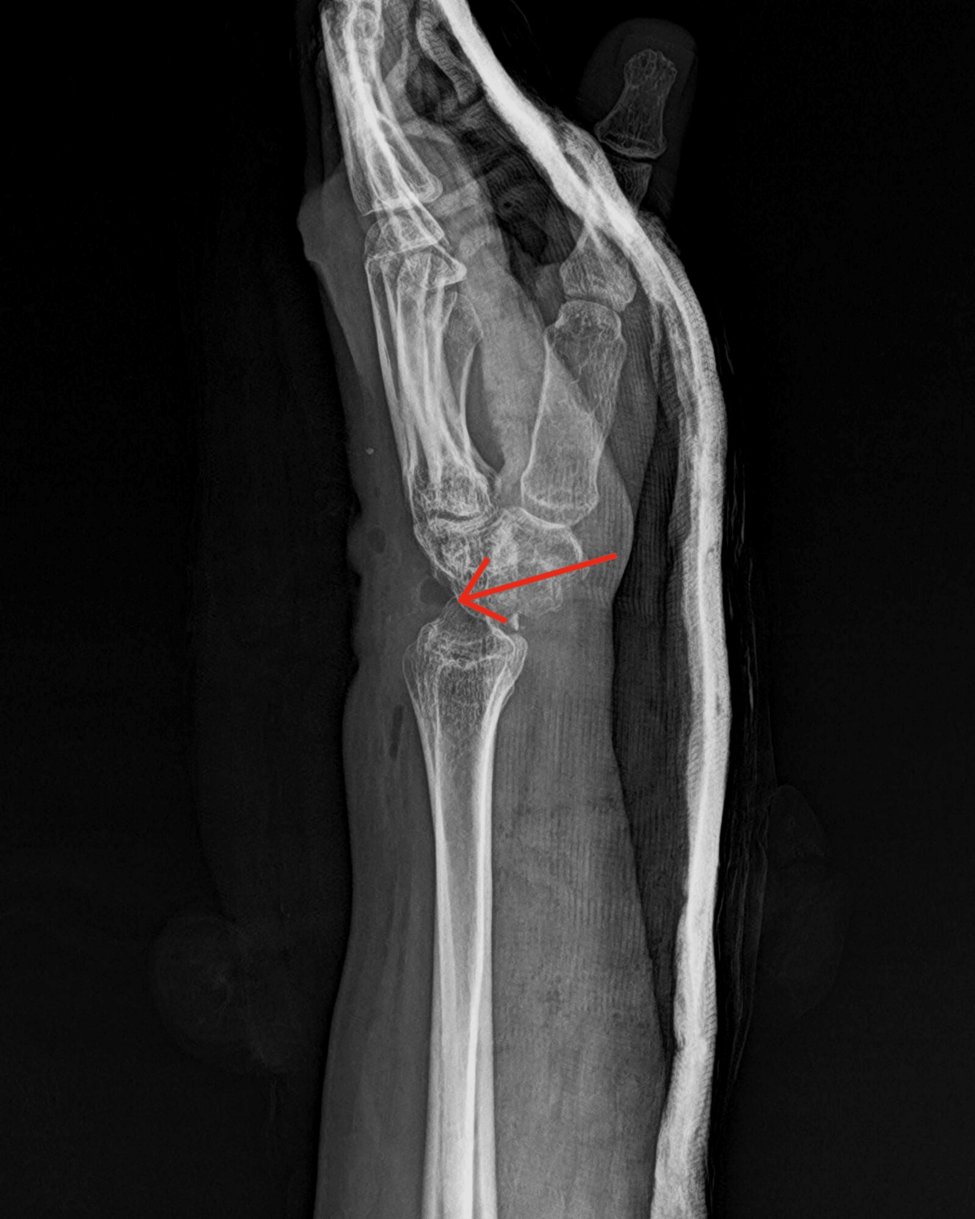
Post-operative radiological findings The plain X-ray in the lateral view shows the absence of the proximal carpal bones, likely due to a partial carpectomy.
